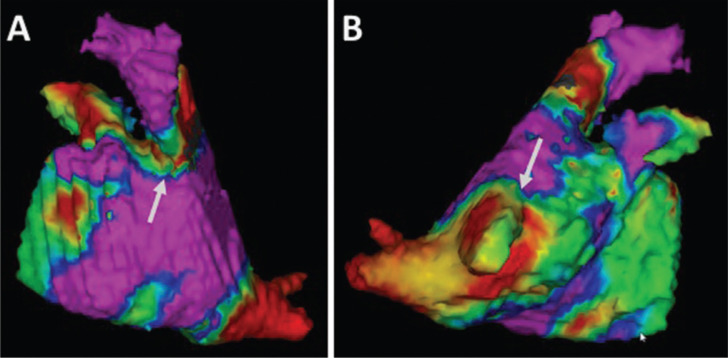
Color projection of a voltage map onto a segmented and registered magnetic resonance imaging of the left atrium. This map represents endocardial peak-to-peak bipolar voltage (color scale from 0.3–1.5 mV, red-yellow-green-blue-purple) created with a high density 20 pole-mapping catheter (PentaRay^®^, Biosense Webster, Inc., Diamond Bar, CA, USA). It reveals gaps of incomplete ablation (arrows). Low voltage signals in the superior portion of the right inferior pulmonary vein in this image were determined to be far field.
